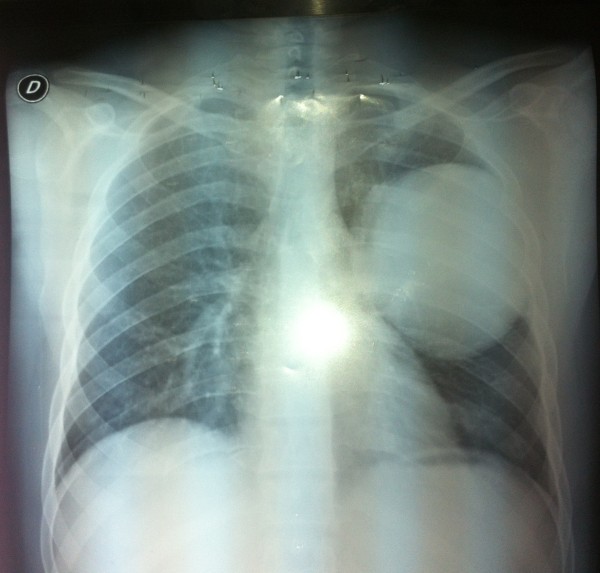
Chest X-ray showing a large left pulmonary opacity.
